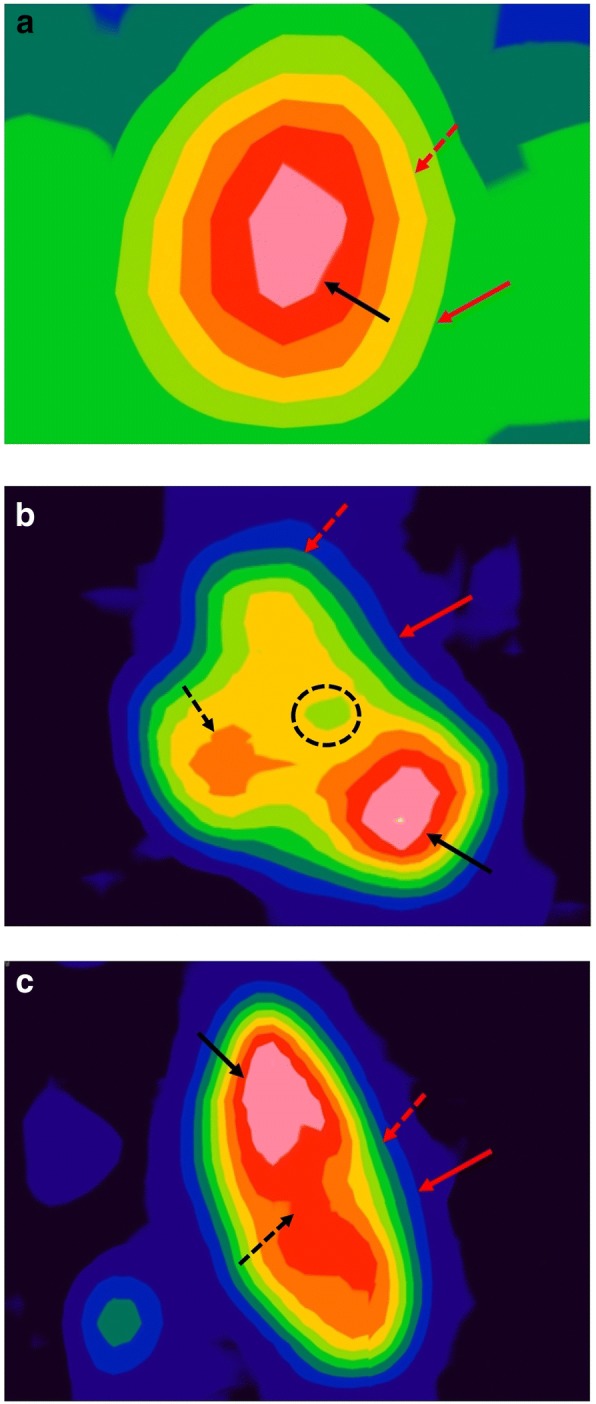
Visual patterns of the tumors. a Visually homogeneous pattern. The hottest core (black arrow) was located in the center of the ESCC. There were no significant irregular layers in the tumor. PHL (50–60% of SUVmax; yellowish green layer) was located between the tumor and the background activity (red arrow). PHL tumor threshold (red dotted arrow) was determined to be 60% of SUVmax (i.e., the innermost portion of the PHL). b Visually heterogeneous pattern. The hottest core was eccentrically located in the ESCC, and a small cold defect was visible (small yellowish green portion in black dotted circle). An irregular layer was also present (black dotted arrow). PHL (20–30% of SUVmax; light blue layer) was located between the tumor and the background activity (red arrow). PHL tumor threshold (red dotted arrow) was determined to be 30% of SUVmax (i.e., the innermost portion of the PHL). c Visually heterogeneous pattern. The hottest core was eccentrically located in the ESCC (black arrow). A prominent irregular layer was present (black dotted arrow). PHL (20–30% of SUVmax; light blue layer) was located between the tumor and the background activity (red arrow). PHL tumor threshold (red dotted arrow) was determined to be 30% of SUVmax (i.e., the innermost portion of the PHL)
